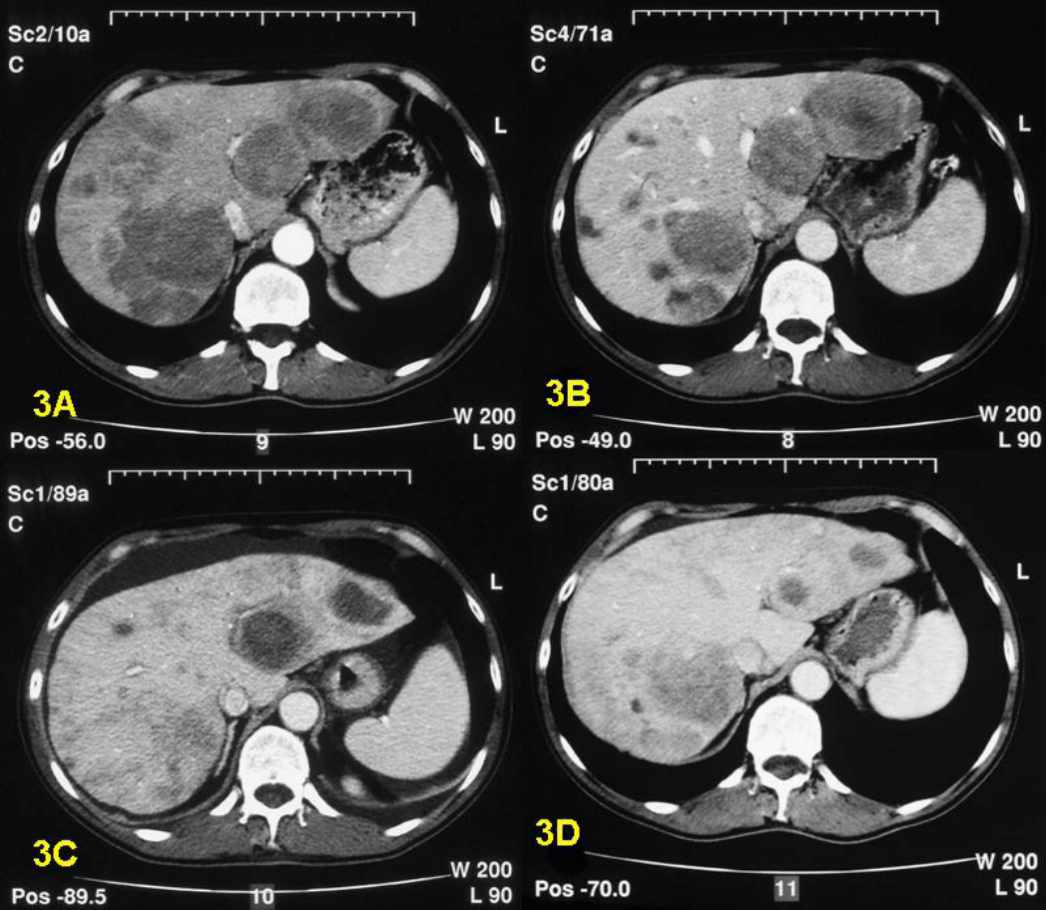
CT-scan of patient 13 reveals several small and larger metastases in both liver lobes (figure 3A). HAE of the right liver lobe resulted in fair tumor reduction (figure 3B). Two large metastases in the left liver lober were treated by RFA and show characteristic cystic appearance (figure 3C). The end result six months after the RFA treatment shows a significant decrease of tumor mass in both liver lobes (figure 3D).
